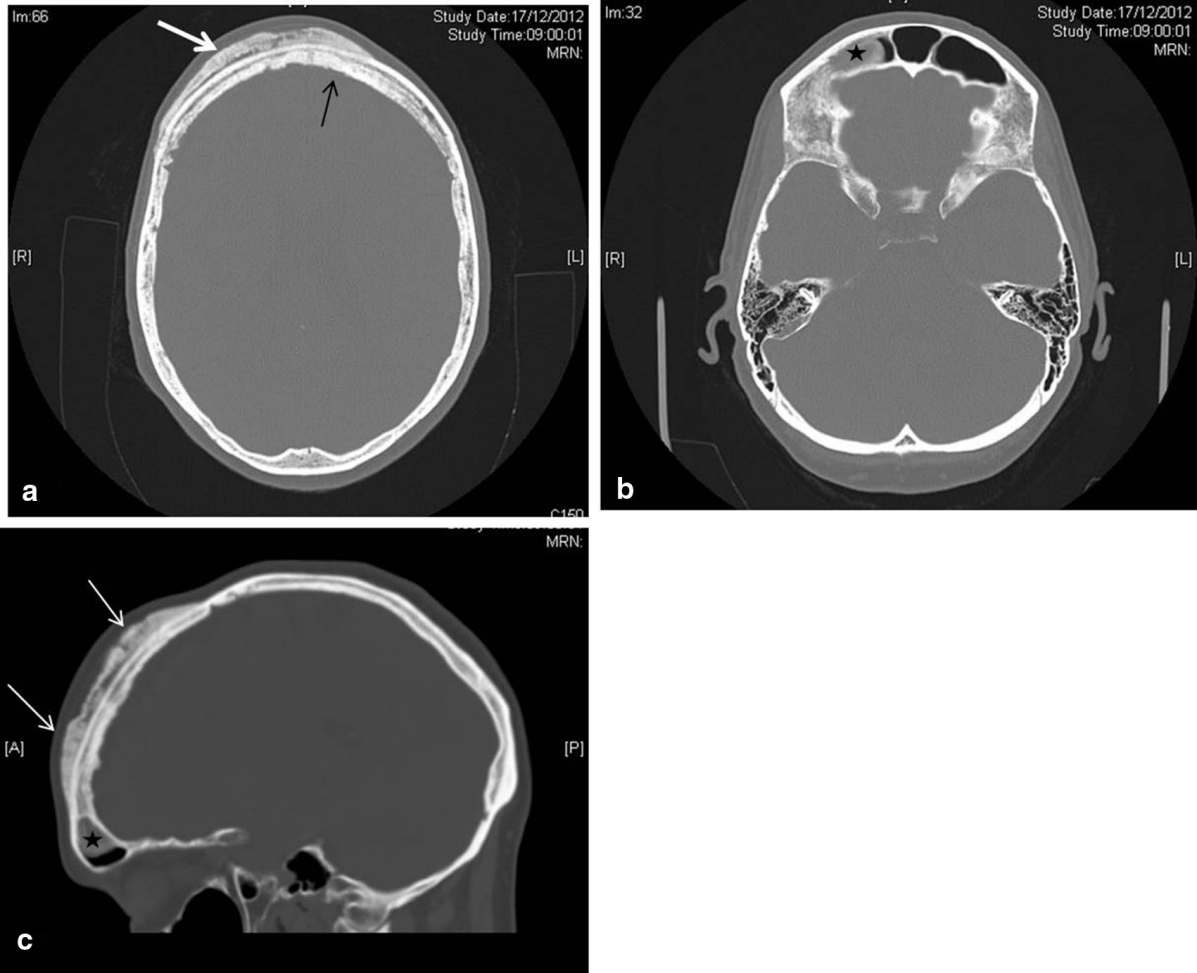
a CT axial image shows a primarily sclerotic lesion involving the diploe (*white arrow*) extending across the midline (*black arrow*). b There is a polypoid mass in the right frontal sinus (*asterisk*). c Sagittal reconstruction shows the lesion (*white arrows*) and the polypoid mass (*asterisk*)
